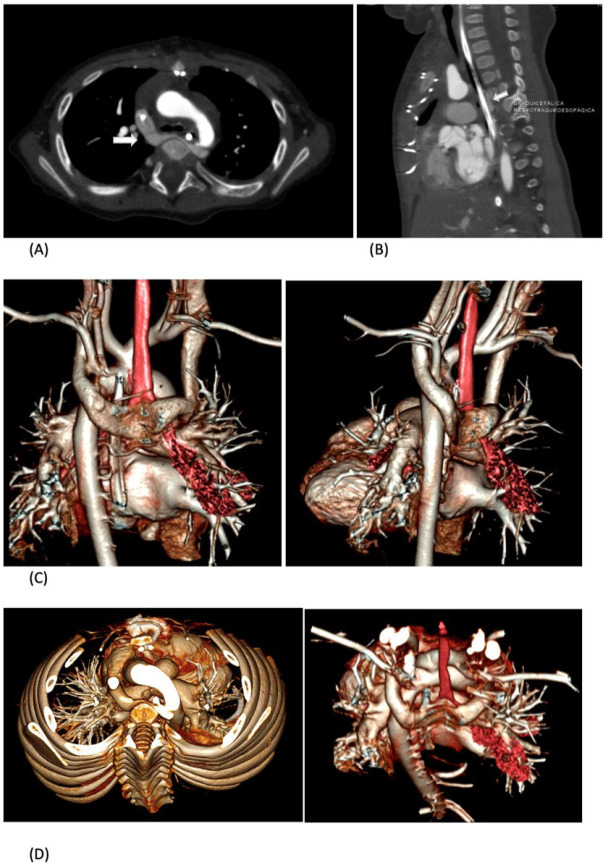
Contrast-enhanced computed tomography showing axial (A) and sagittal
